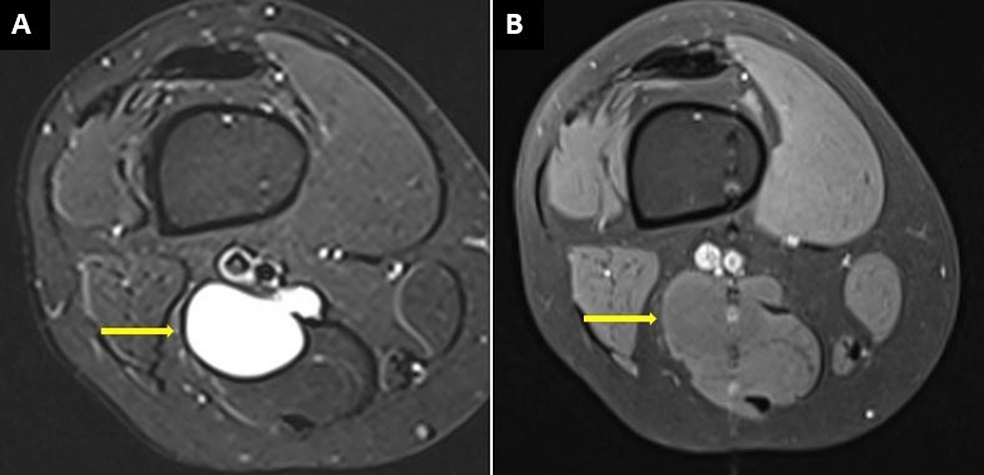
MRI of the right thigh (A) Axial-STIR sequence demonstrating a well-defined high signal intensity lesion originating from the semimembranosus tendon. (B) On the post-contrast sequence, there is minimal rim enhancement with no remarkable internal enhancement of the lesion.
